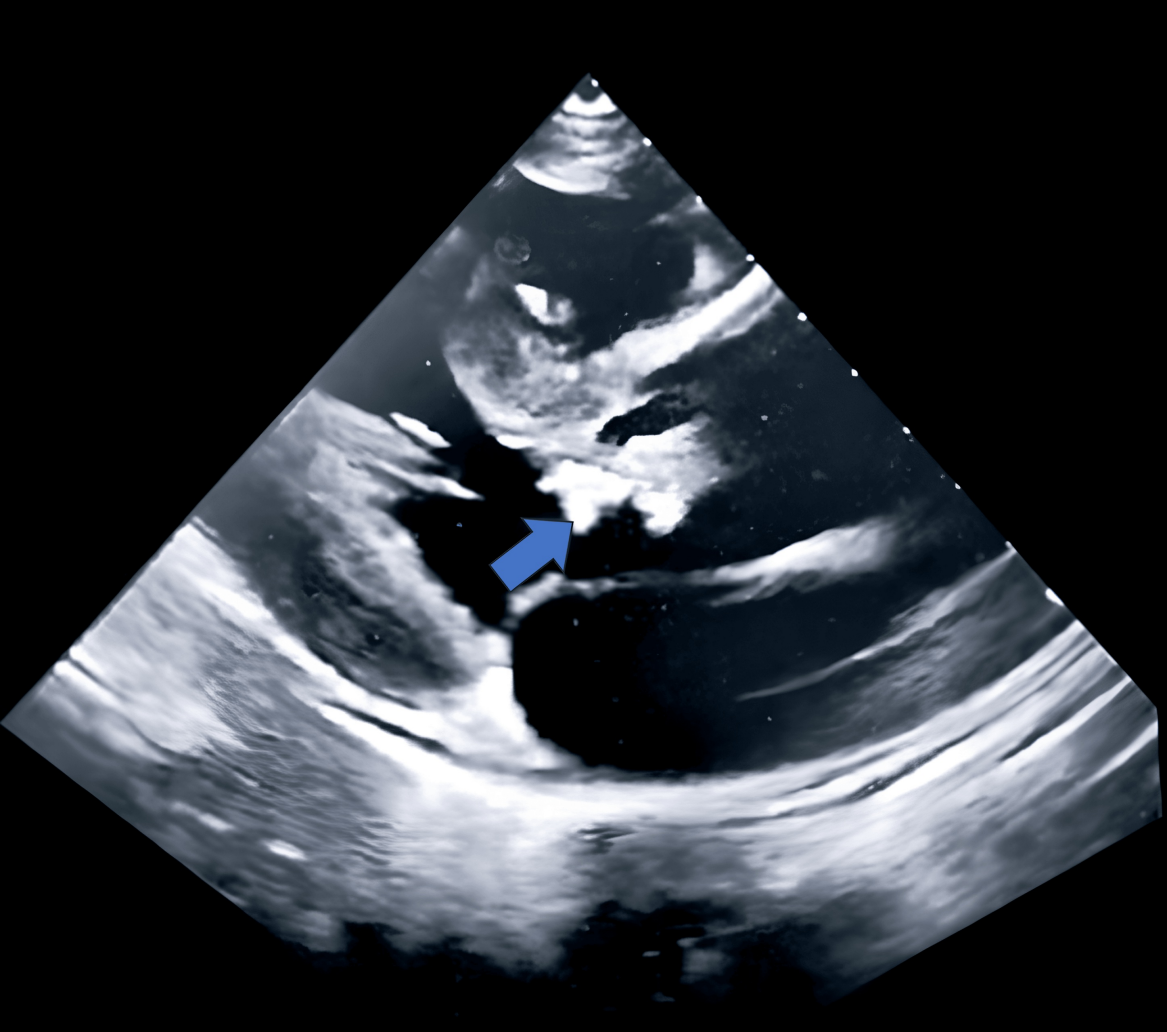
Two-dimensional parasternal long-axis echocardiogram showing large vegetation on the aortic valve (blue arrow)
